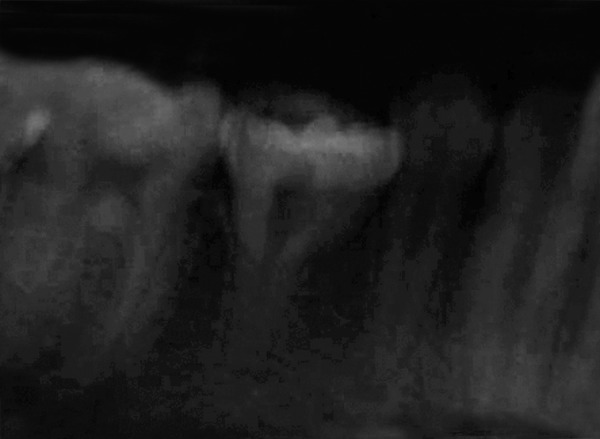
Intraoral periapical radiograph of the patient depicting unusual morphology of mandibular left second premolar
